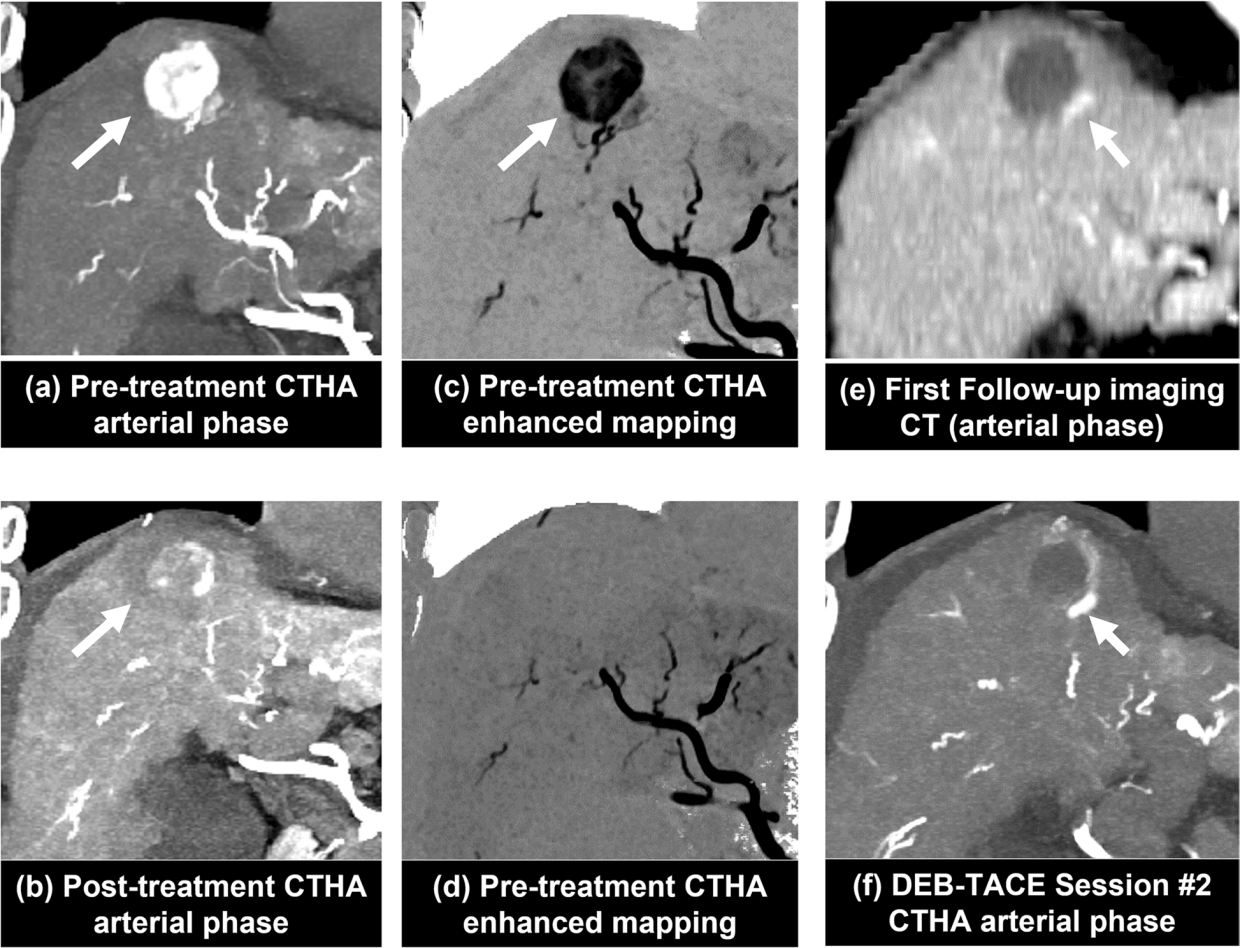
A false-negative case for no-residual enhancement on CTHA-EM analysis showing residual tumor on first follow-up imaging 13.3 weeks later. a Pretreatment CTHA arterial phase showed hypervascular HCC (white arrow). b Posttreatment CTHA showed retention of contrast media in the tumor (white arrow). c Pre-treatment CTHA-EM image shown in inverted gray scale with HCC (white arrow). d Posttreatment CTHA-EM demonstrated lack of residual tumor enhancement at the target HCC or its feeding artery, therefore identified as “no-residual.” e First follow-up CT imaging (arterial phase) showed residual tumor (white arrow). f Second session of pretreatment CTHA confirmed residual tumor along the border of treated tumor (white arrow). Tumor progression instead of residual untreated tumor cannot be excluded due to long follow-up period from DEB-TACE procedure to first follow-up imaging. *CTHA*, Computed tomography hepatic arteriography; *CTHA-EM*, CTHA enhancement mapping; *DEB-TACE*, Drug-eluting beads transarterial chemoembolization; *HCC*, Hepatocellular carcinoma
